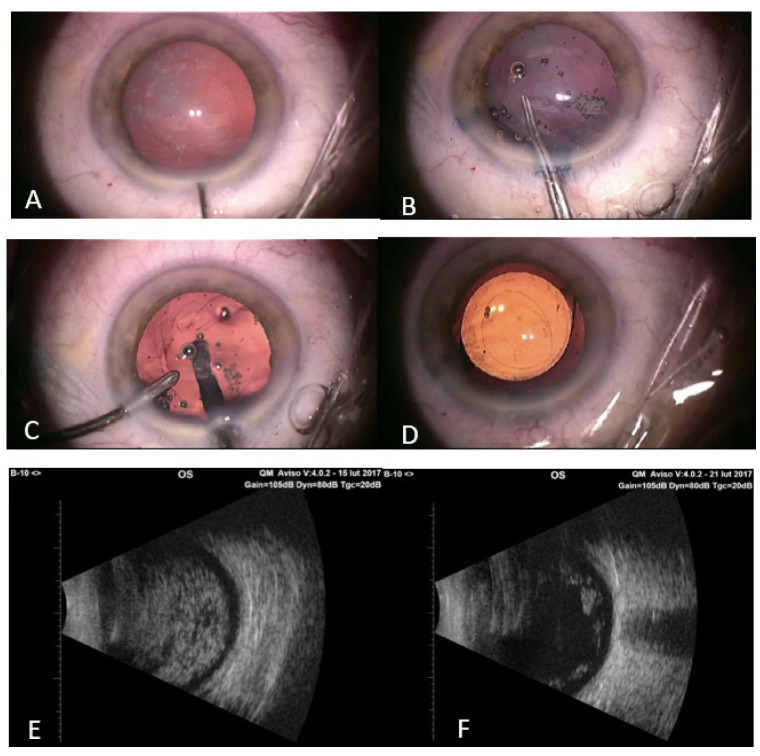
(A) Patient’s eye after phacoemulsification. (B) Posterior capsulorhexis with capsulorhexis forceps. (C) Twenty-gauge vitreous cutter introduced to the vitreous cavity behind the posterior capsule, AC maintainer introduced through the sideport to the anterior chamber. (D) Final result after PCIOL implantation. (E) Patient’s ultrasound B scan before surgery. (F) The same patient’s ultrasound B scan after surgery.
